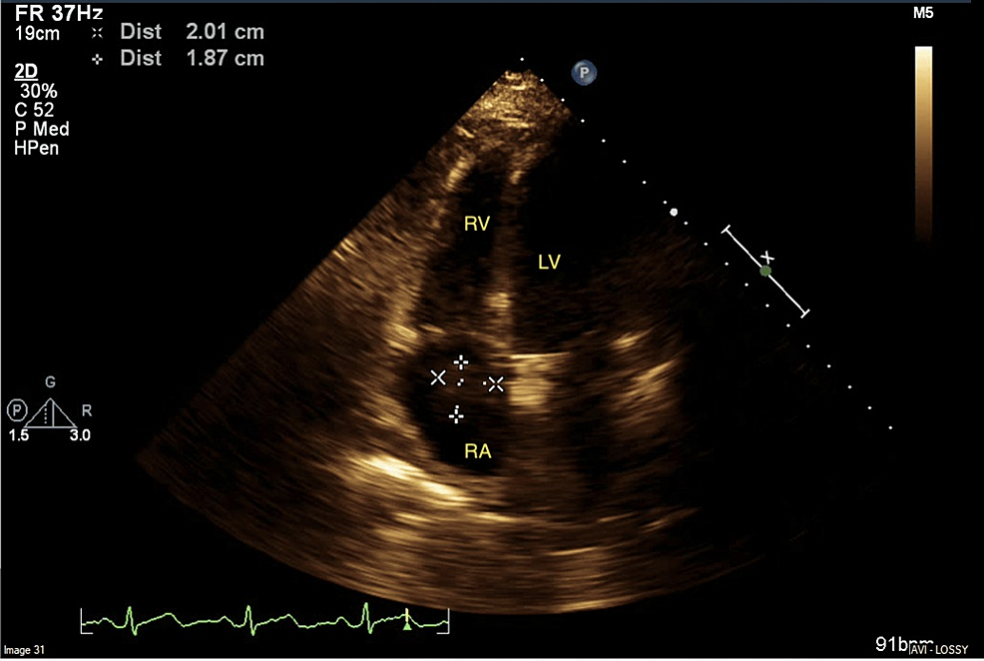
Right atrial mass seen in the apical 4 chamber view RA: right atrium; RV: right ventricle; LV: left ventricle
